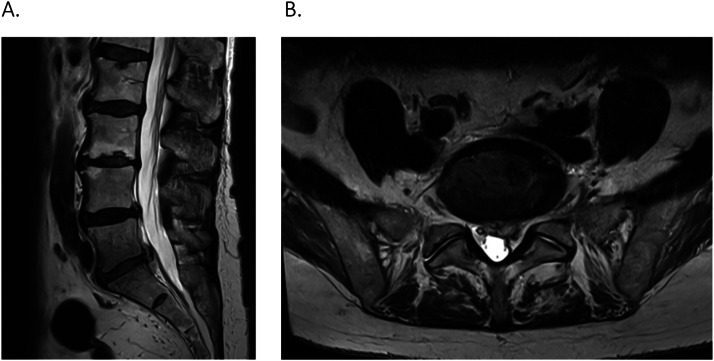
(A) T-2 weighted MRI L-spine on sagittal view demonstrates the patient's initial disc budge at L5/S1. (B) T-2 weighted MRI L-spine on axial view of the L5/S1 disc with left paracentral disc protrusion and compression of left S1 nerve root.
